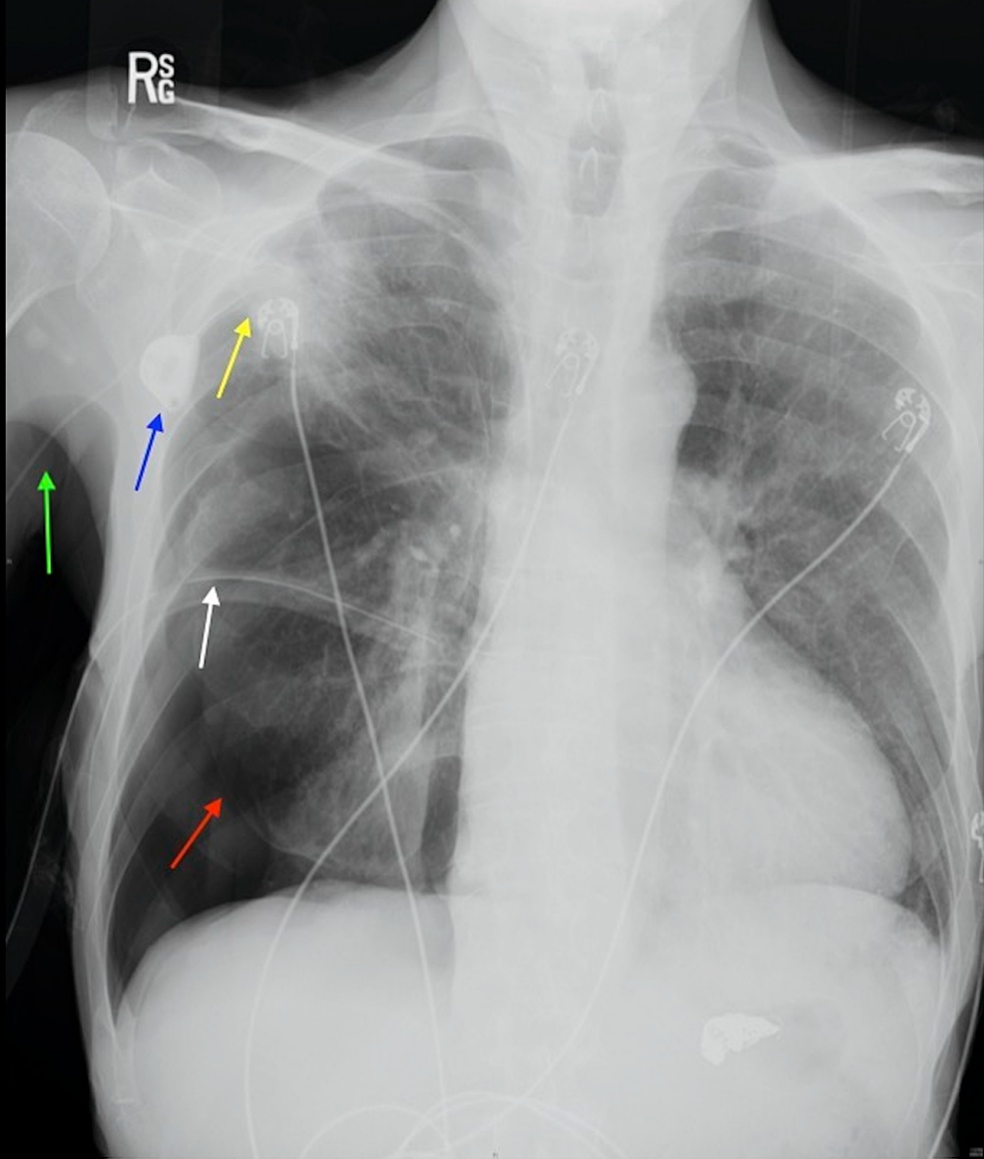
Chest radiograph demonstrating a right-sided pneumothorax (red arrow), a right-sided apical cavitary lesion (yellow arrow), a chemotherapy port (blue arrow), a PICC line (green arrow), and a chest tube (white arrow). PICC: peripherally inserted central catheter
